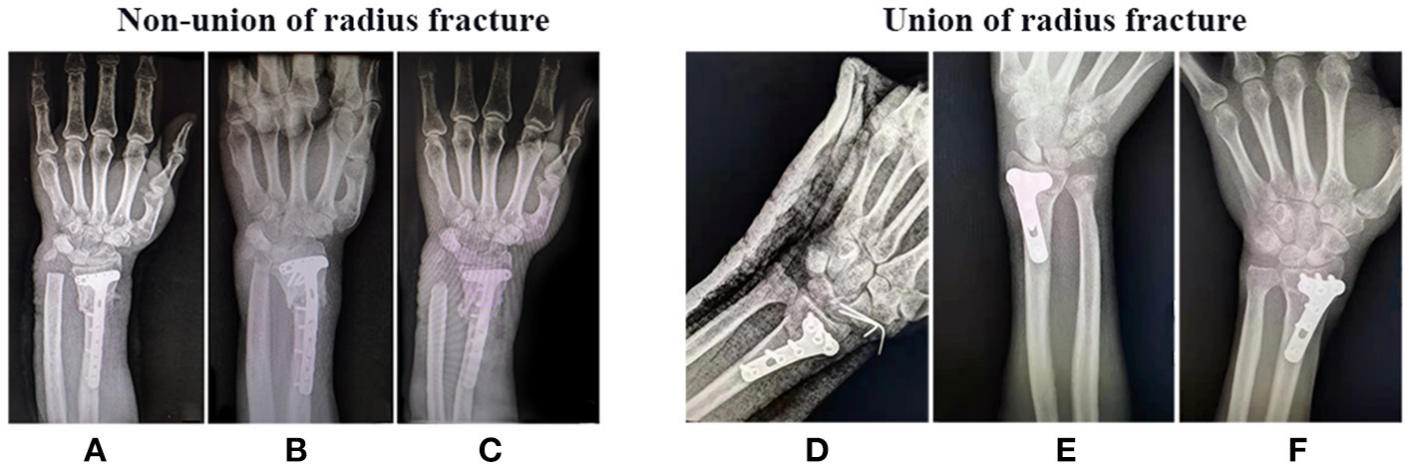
Non-union and Union of radius fracture after surgery. X-ray examination (A,D) 1 month, (B,E) 3 month, and (C,F) 9 month after surgery.
